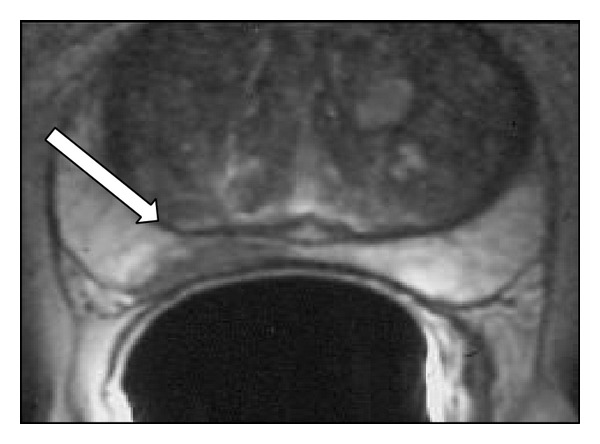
The presurgical high resolution T2w MRI imaging with a dark intraprostatic hypointense spot (white arrow) in the context of the right lobe relatively hyperintense peripheral zone, demonstrating an apparently organ-confined neoplastic disease.
